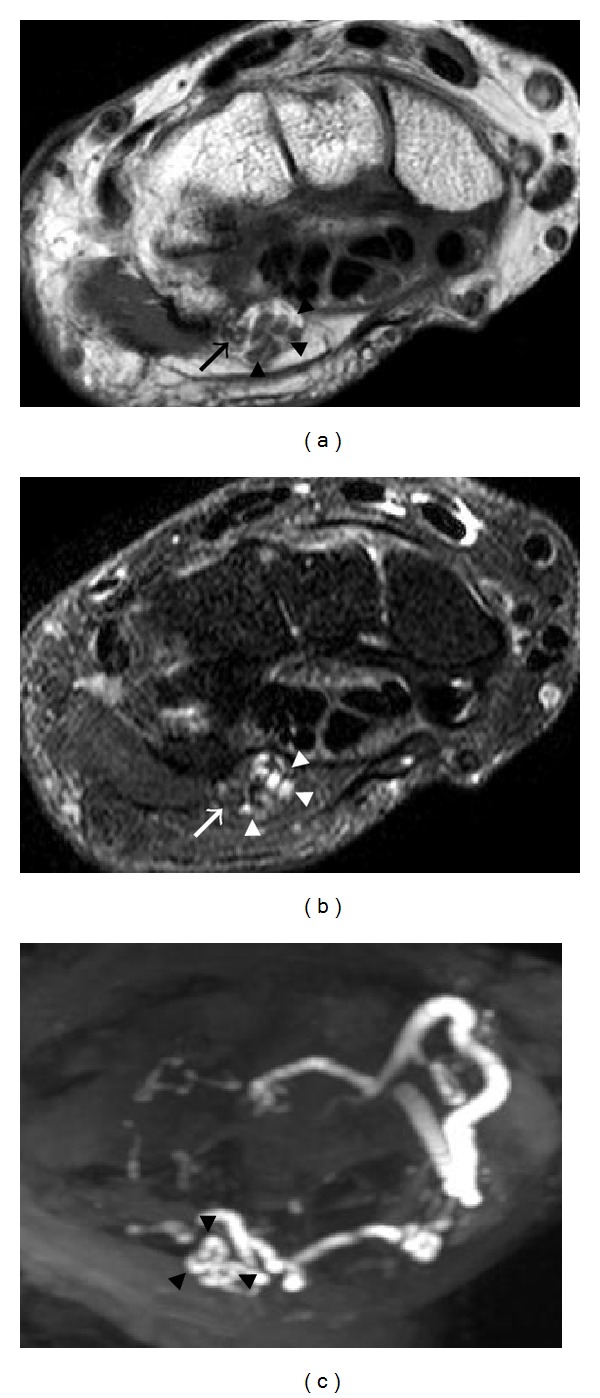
A 73-year-old patient with clinical evidence of right ulnar nerve compression at wrist. Axial T1-weighted (a), T2-weighted with fat saturation (b), and axial SENSE MIPs (c) identify the ulnar nerve (arrows) in a crowded Guyon's canal compressed by a tortuous ulnar artery (arrowheads).
